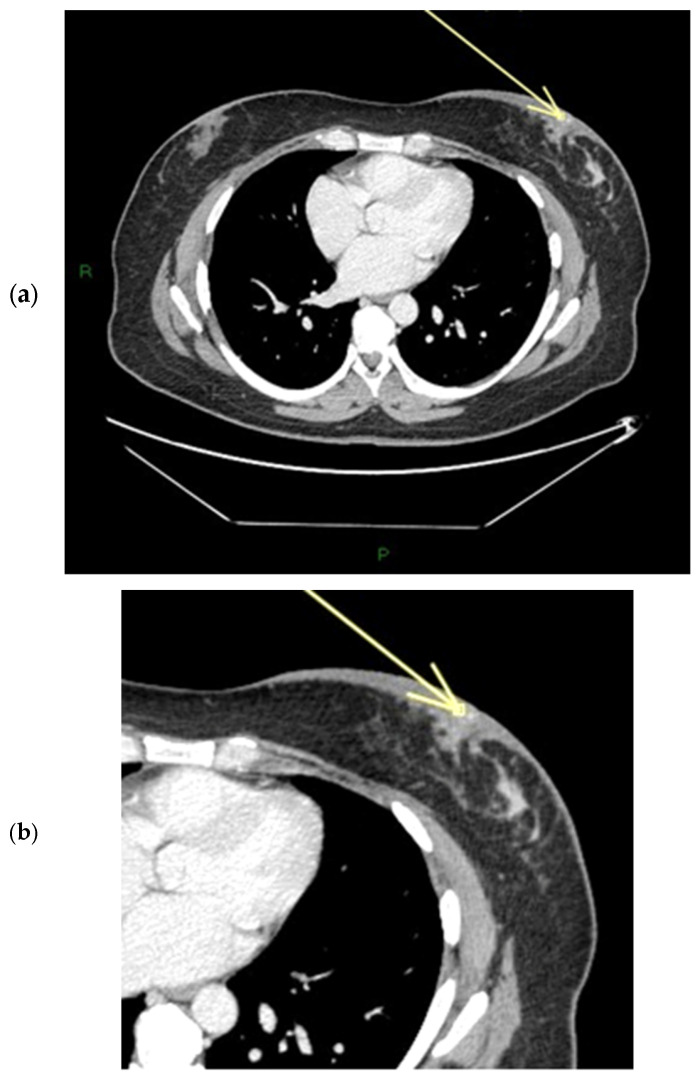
(a,b) Patient’s name R.M. In the ce-CT scan, the arrow shows enhancement of the base of the left nipple alone (and not of the body).
